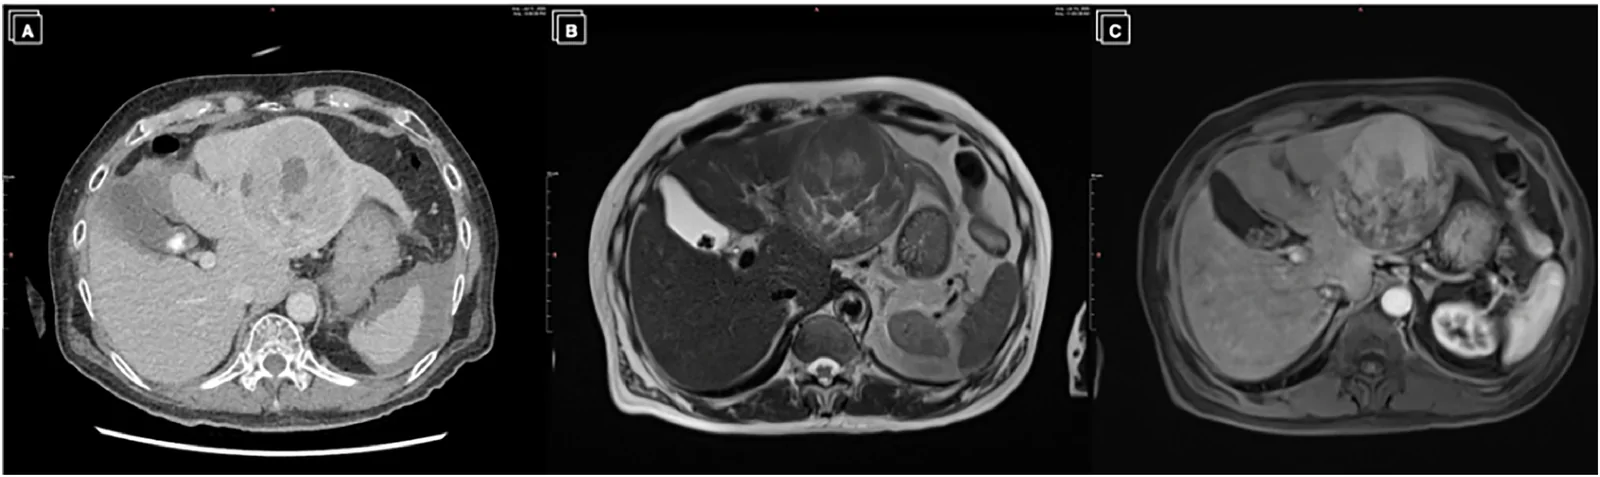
Pretreatment CT and MRI evaluation of a hemorrhagic hypervascular left hepatic lobe mass. (A) Contrast-enhanced CT venous phase obtained July 11 demonstrates a large heterogeneous mass centered in the left hepatic lobe with associated hemoperitoneum. (B) axial T2-weighted HASTE MRI obtained July 14 demonstrates internal heterogeneity within the mass, compatible with hemorrhagic and necrotic components. (C) Arterial-phase postcontrast T1-weighted VIBE MRI obtained July 14 demonstrates prominent hypervascular enhancement within the mass. Overall imaging findings raised concern for hemorrhagic hepatocellular carcinoma, later confirmed by biopsy.
